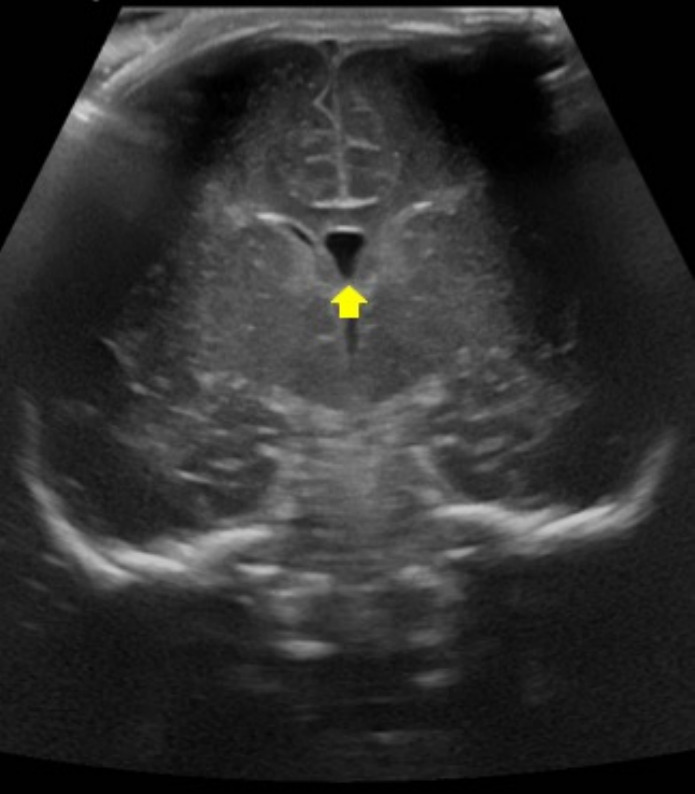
Cerebral ultrasound of 1-day-old premature male born at 32 weeks and 4 days of gestation showed midline fluid-filled cyst between the lateral ventricles on the coronal plane (yellow arrow) consisting in common anatomical variant such as Cavum Septum Pellucidum
